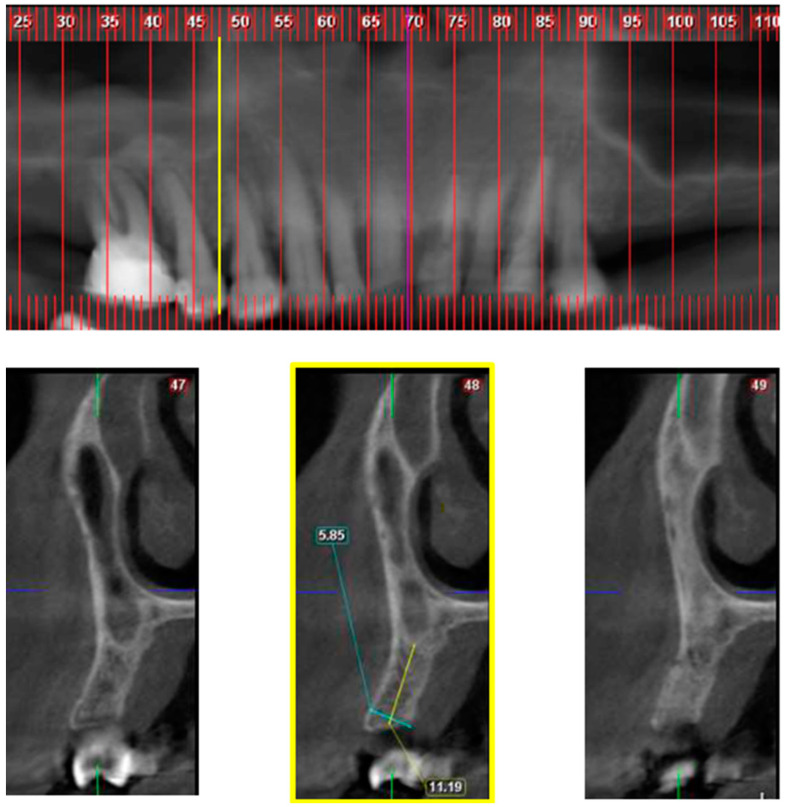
Class 2; Upper part—CBCT panoramic reconstruction; Lower part—from right to left—CBCT cross sections: cross section number 49—demonstrate nasal cavity alone above the alveolar ridge; cross section number 48—yellow line and frame—demonstrate nasal and maxillary sinus “border cross section”, which is located between 1st and 2nd premolars, defined as Class 2; cross section number 47—demonstrate one cross section distal to the “border cross section”.
